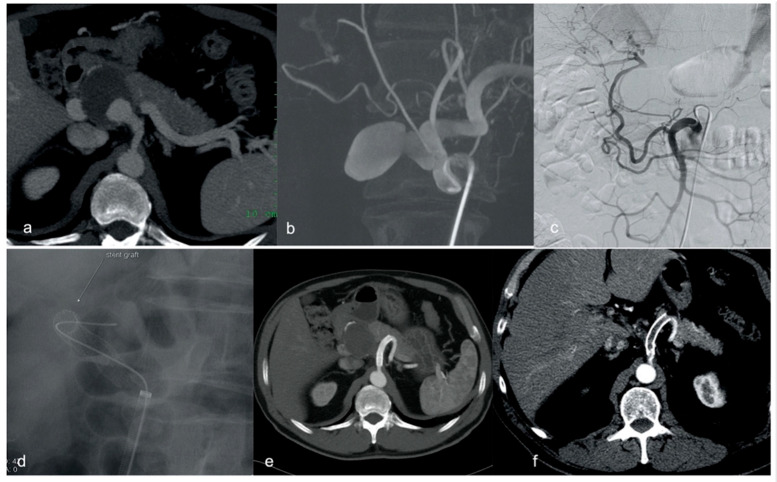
Multimodal imaging and long-term follow-up of a hepatic pseudoaneurysm. (a) Angio CT shows a pseudoaneurysm of the hepatic artery origin with dissection and obstruction of the artery; (b) dynamic CT angiography used for pre-treatment planning; (c) angiogram of the superior mesenteric artery demonstrating collateral flow to the distal hepatic artery; (d) intraoperative image of Viabahn stent graft deployment in the coeliac trunk and in the splenic artery to exclude the aneurismatic origin of the hepatic artery; (e) CT at 5 years with patency of the implanted stent graft; (f) occluded stent graft at 139 months follow-up.
